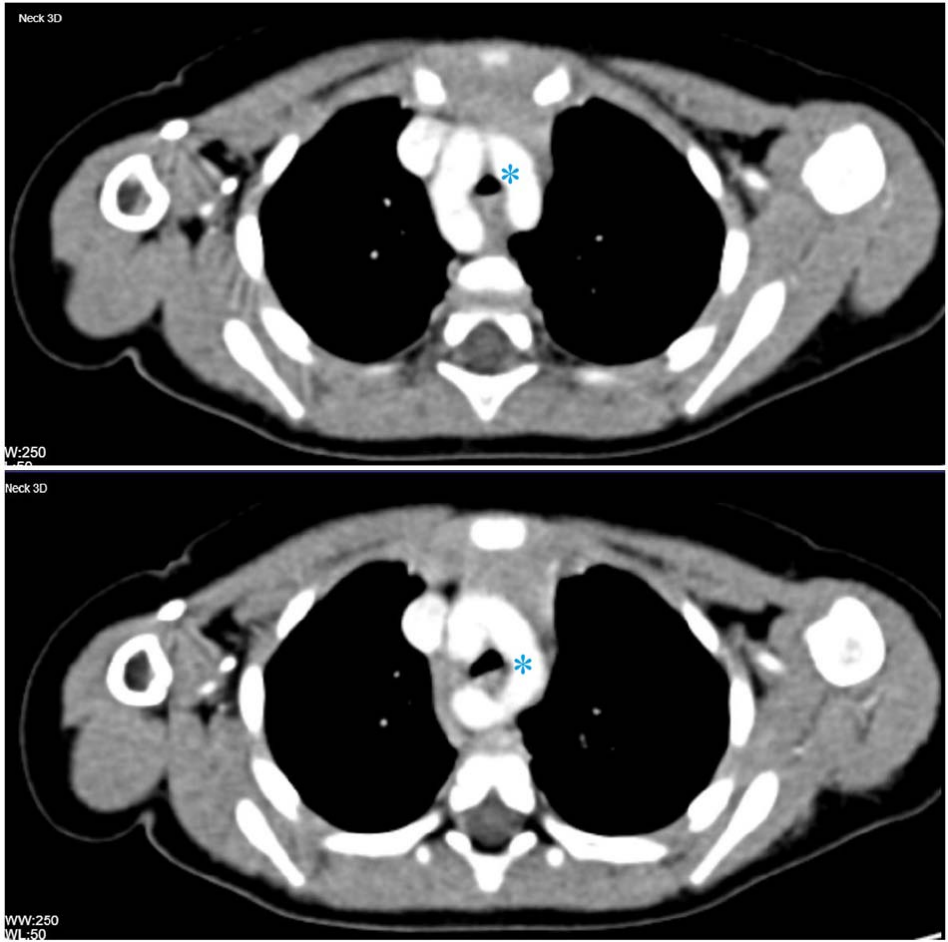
Contrast-enhanced CT of the chest in two consecutive axial cuts showing duplication of the aortic arch encircling the thoracic trachea and oesophagus (asterisk).
